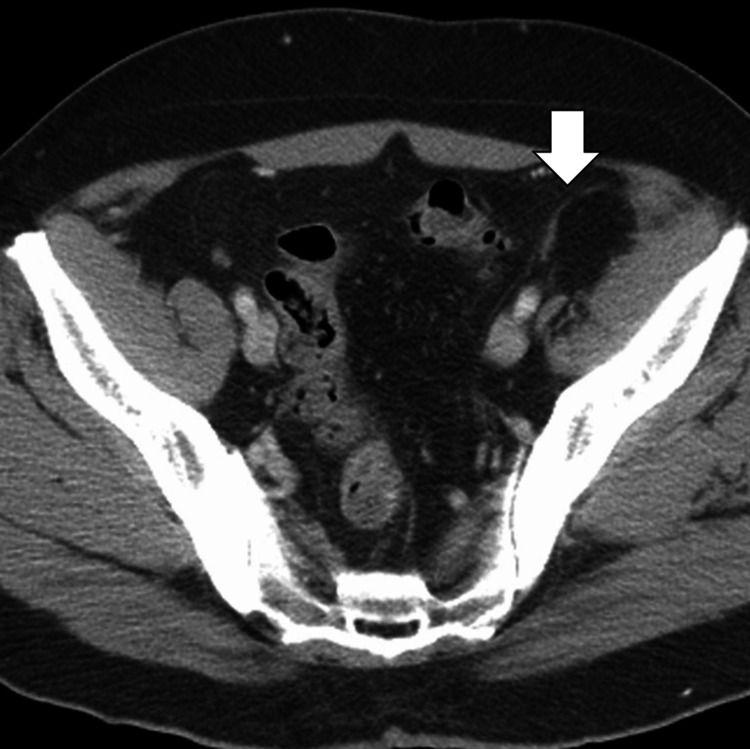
Axial contrast-enhanced CT image of the abdomen demonstrating epiploic appendagitis (arrow) adjacent to the descending colon. Note the characteristic fat-stranding and the "central dot" sign, representing a thrombosed vessel within the inflamed appendage.
